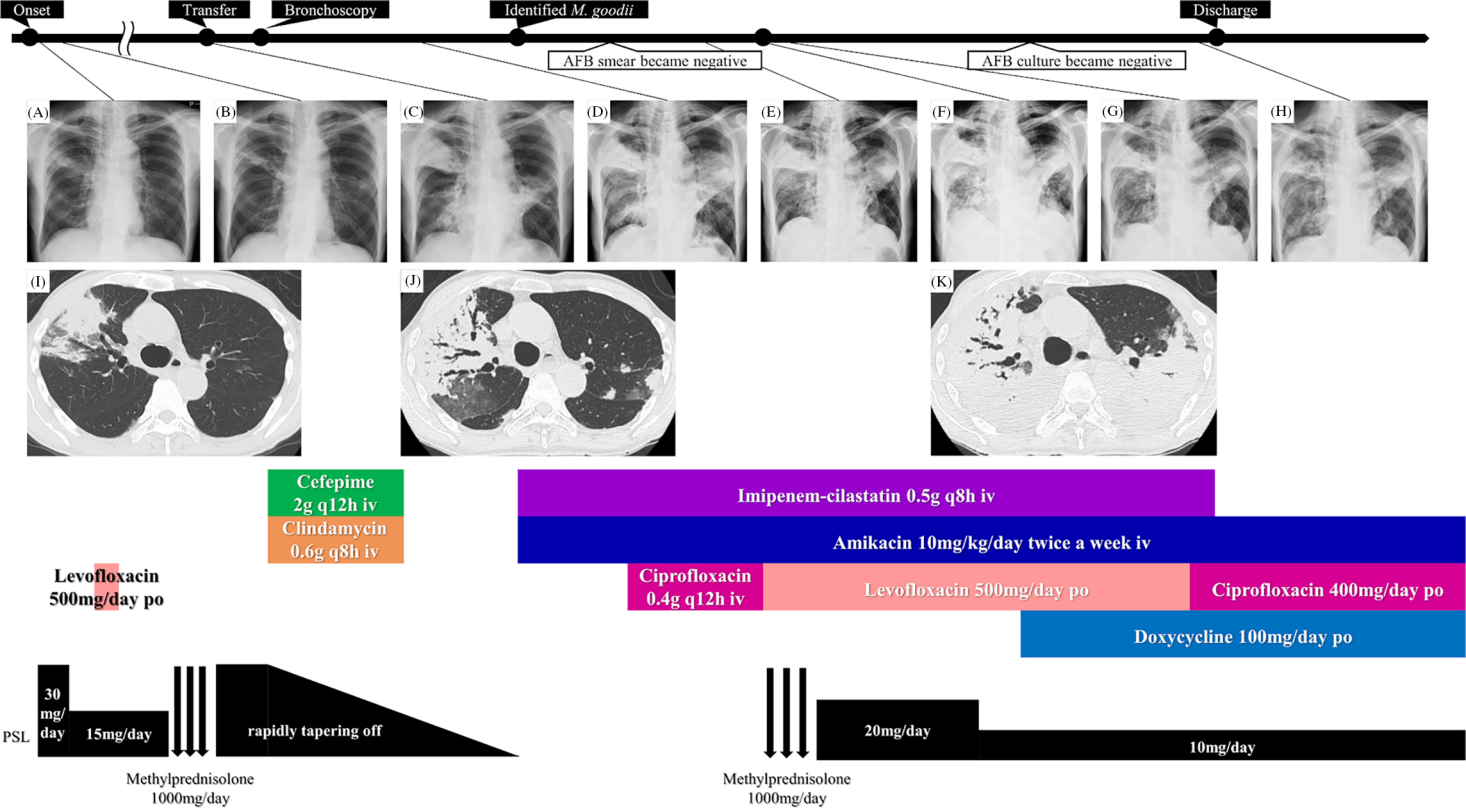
Imaging findings of chest radiography and treatments over time. (A) Chest radiography reveals consolidation in the right middle lung when symptoms developed. (B) Chest radiography reveals improvement in consolidations in the right middle lung after initiating prednisolone (30 mg/day) treatment. (C) Chest radiography reveals exacerbation of bilateral consolidations despite administration of antibiotics and methylprednisolone (1000 mg/day for 3 days). (D) Chest radiography reveals mild exacerbation of bilateral consolidations after administration of antibiotics. (E) Chest radiography reveals improvement in bilateral consolidations after initiating antimycobacterial therapy. (F) Chest radiography reveals acute exacerbation of bilateral consolidations on day 29 after hospitalization. (G) Chest radiography reveals improvement in bilateral consolidations after administration of methylprednisolone (1000 mg/day for 3 days). (H) Chest radiography reveals improvement in bilateral consolidations before discharge. (I) Chest computed tomography (CT) reveals consolidation in the right upper lobe of the lung when symptoms developed. (J) Chest CT reveals exacerbation of the consolidation in the right upper lobe of the lung and development of new consolidations in other lobes of the lung. (K) Chest CT reveals exacerbation of bilateral consolidations on day 29 after hospitalization. AFB, acid‐fast bacilli; *M. goodii*, *Mycobacterium goodii*; po, per os; PSL, prednisolone; iv, intravenous.
